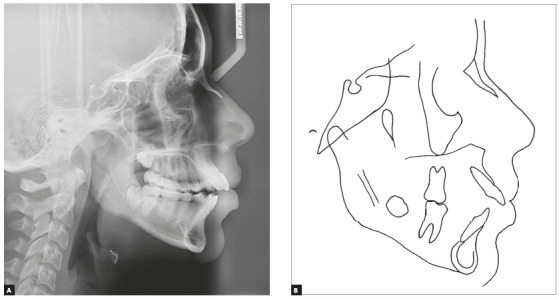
Initial cephalometric radiograph (A) and cephalometric tracing
